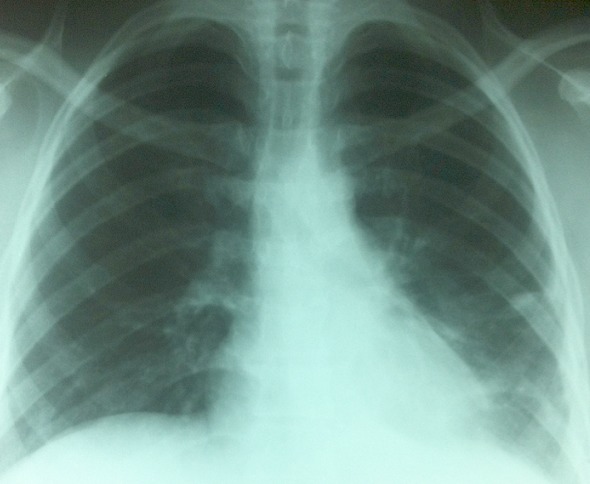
Radiographie thoracique de contrôle après 6 mois de l'intervention
